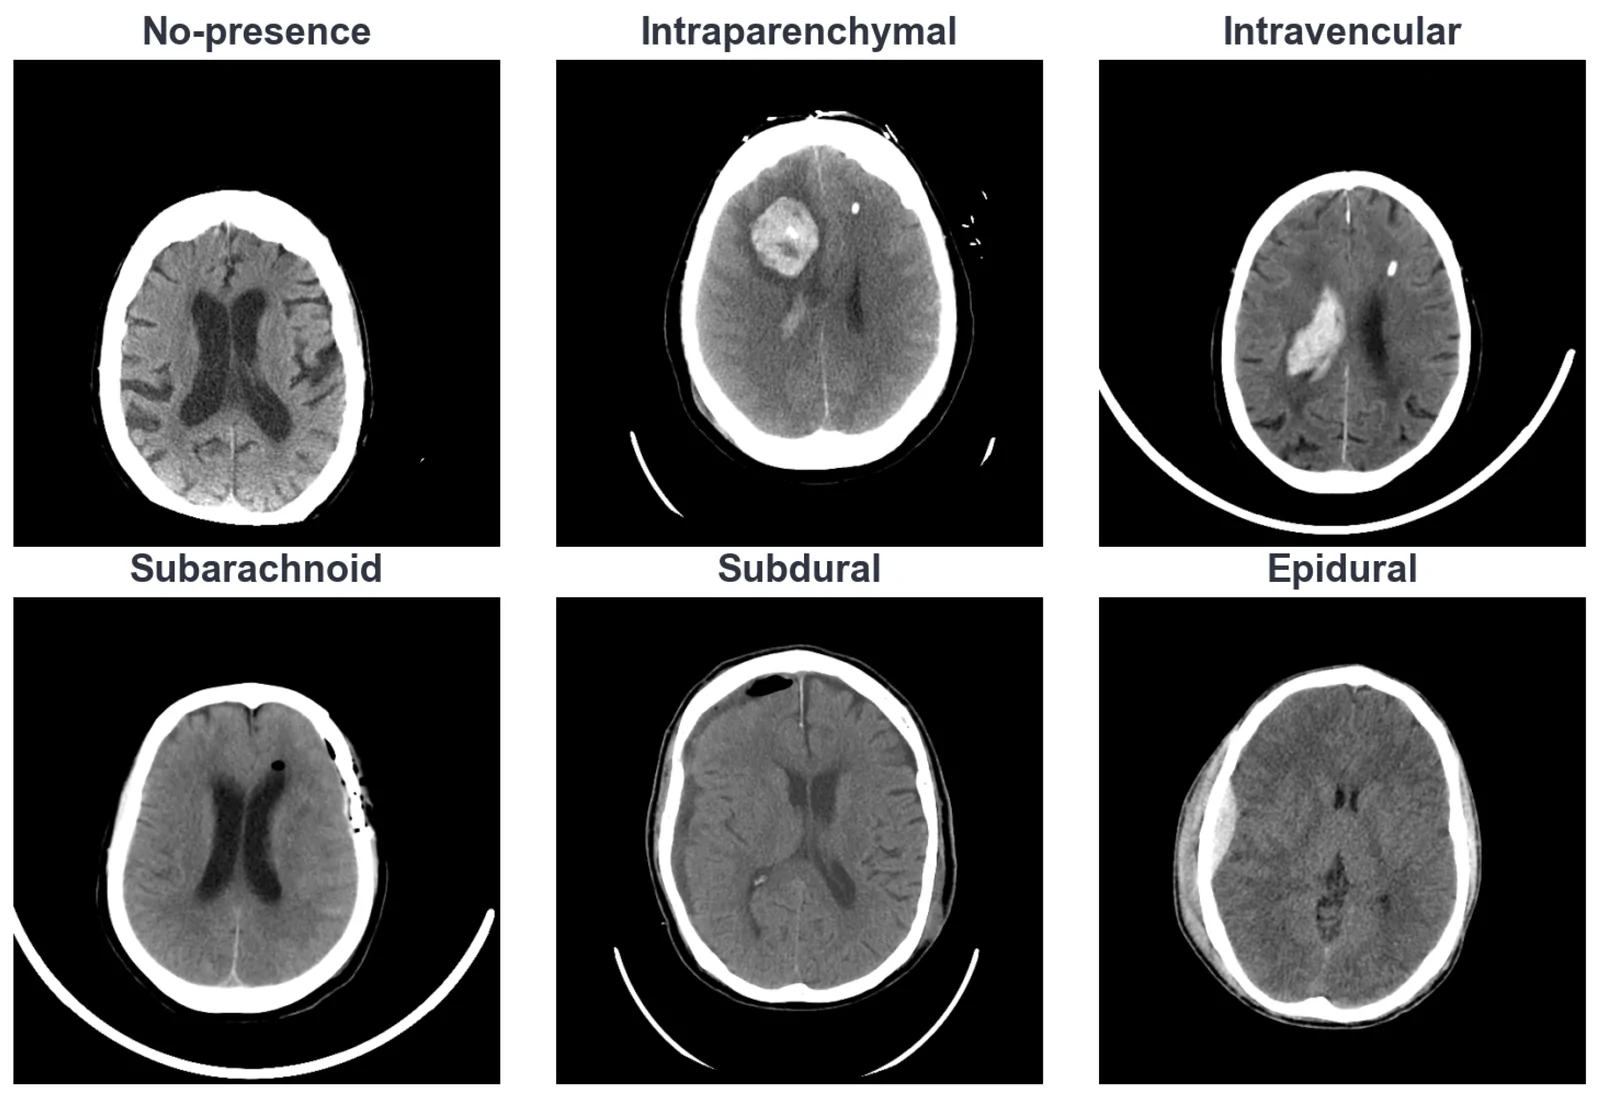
CT slices showing a negative case and the five ICH subtypes: IPH, IVH, SAH, SDH, and EDH.
